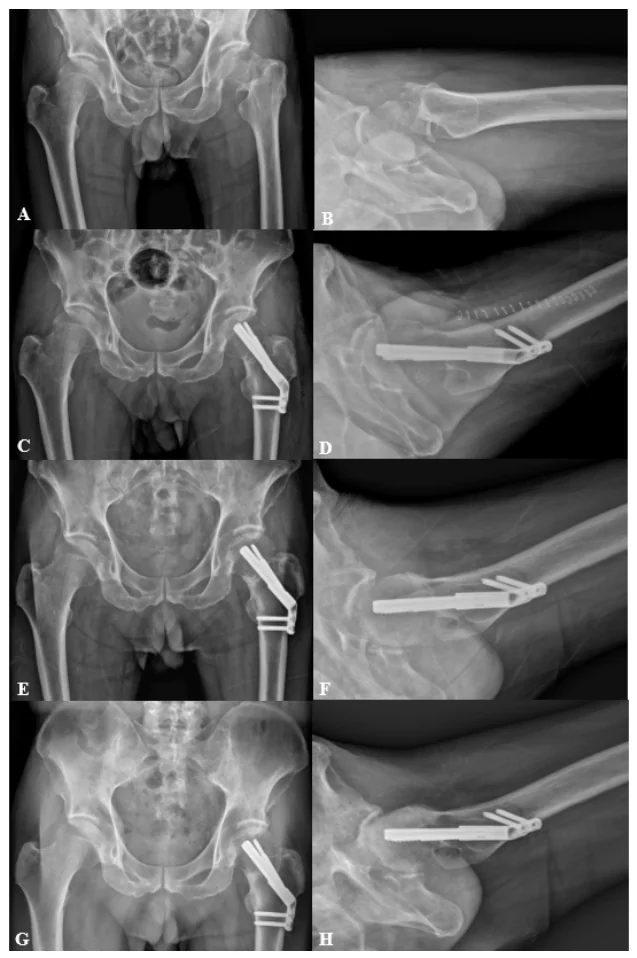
A 61-year-old male sustained a left femoral neck fracture (FNF) following a ground-level fall. Initial anteroposterior (A) and lateral (B) radiographs show a displaced subcapital fracture, classified as Garden stage IV. Closed reduction and internal fixation using the Femoral Neck System (FNS) was performed on the day of injury. Immediate postoperative anteroposterior (C) and lateral (D) radiographs demonstrate satisfactory reduction and implant placement. At six months postoperatively, anteroposterior (E) and lateral (F) radiographs confirm fracture union. Although the patient remained asymptomatic, anteroposterior (G) and lateral (H) radiographs obtained at 18 months postoperatively show subchondral sclerosis and femoral head flattening, consistent with osteonecrosis of the femoral head (ONFH). As the patient remained functional and symptom-free, no revision surgery was performed, and the condition was managed conservatively.
